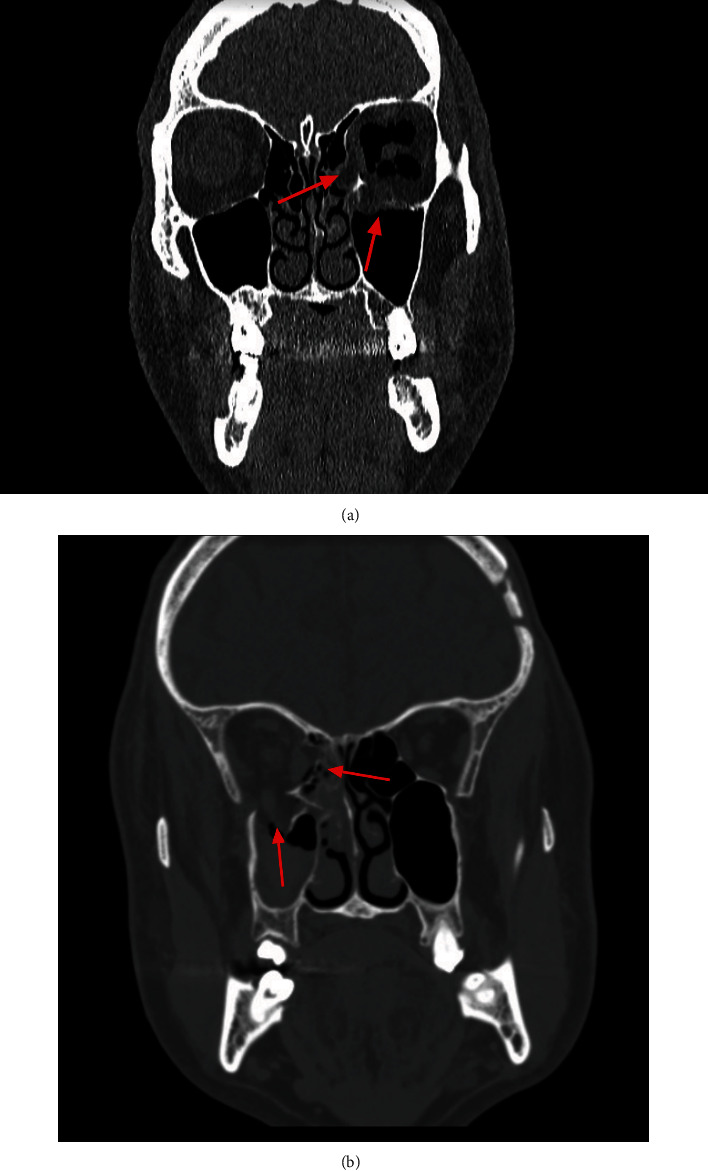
Exemplary CT scan analysis; red arrows indicate observed orbital wall fractures. (a) Male, 49, suffering both eyeball and orbital injury after boxing training with multifracture of the medial and lower walls of the left orbit. Bone fragment dislocated into maxillary and ethmoidal sinuses. (b) Male, 59, a fight victim suffering multifracture orbital trauma including both medial and lower walls of the right orbit with translocation of the bone fragment into maxillary and ethmoidal sinuses. Clinically diagnosed diplopia and damage of maxillary nerve (CN V_2_).
